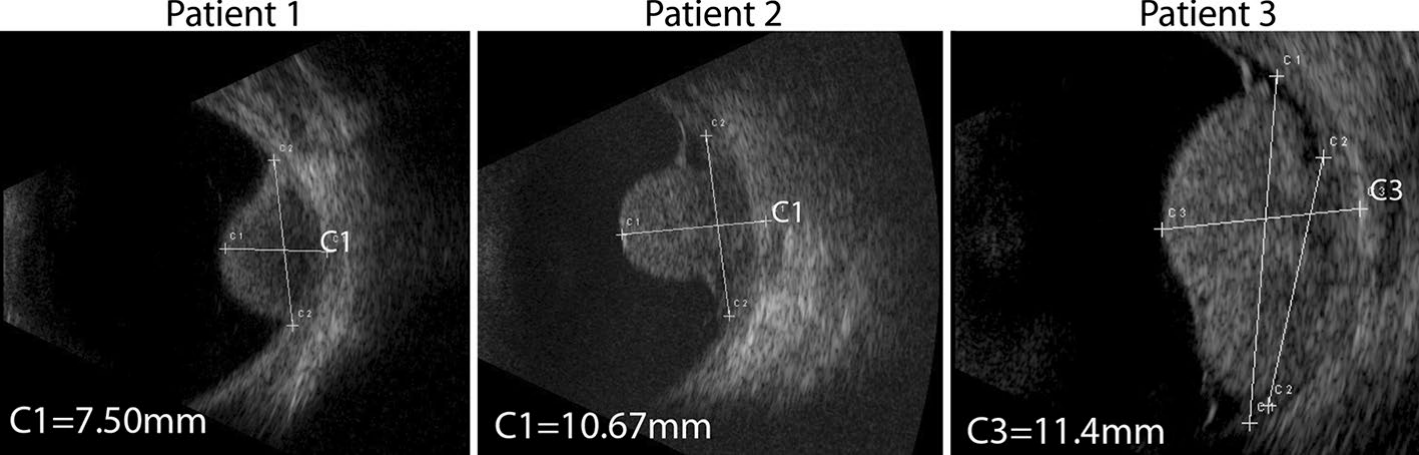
B-scan ultrasonographic images of the eyes of three patients showing uveal melanoma
